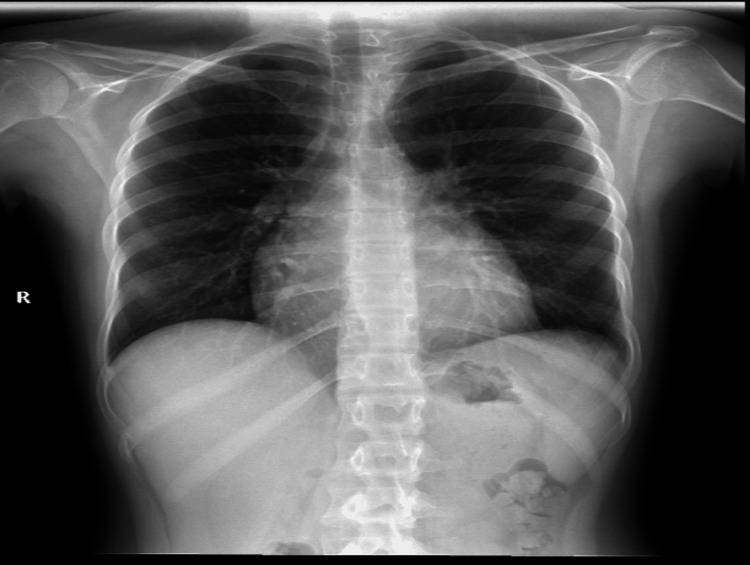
Chest X-ray repeated after five days of pulse steroid therapy and a low dose of diuretics, which shows a huge improvement in comparison to the previous one, in terms of improvement in the pleural effusion and cardiomegaly
